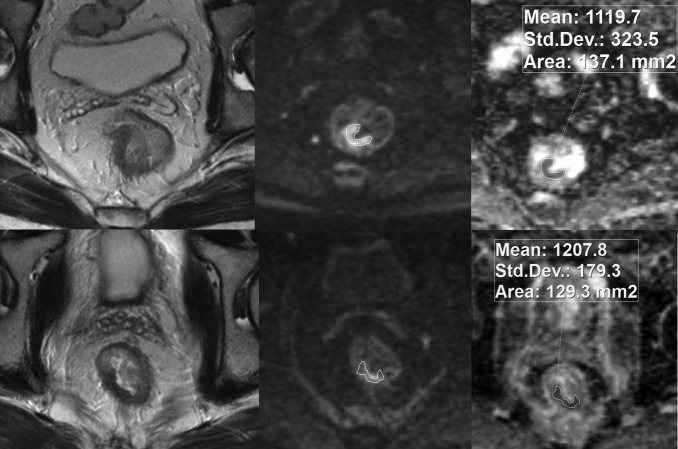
Pre-chemoradiotherapy (CRT) and post-CRT T2-weighted (left column), diffusion-weighted images (middle column), and apparent diffusion coefficient (ADC) (right column) image sets of rectal cancer estimated at pre-CRT magnetic resonance imaging as T3a from patient who experienced good response to CRT (TRG 2). Numbers listed in the ADC images indicate particular regions of interest.
